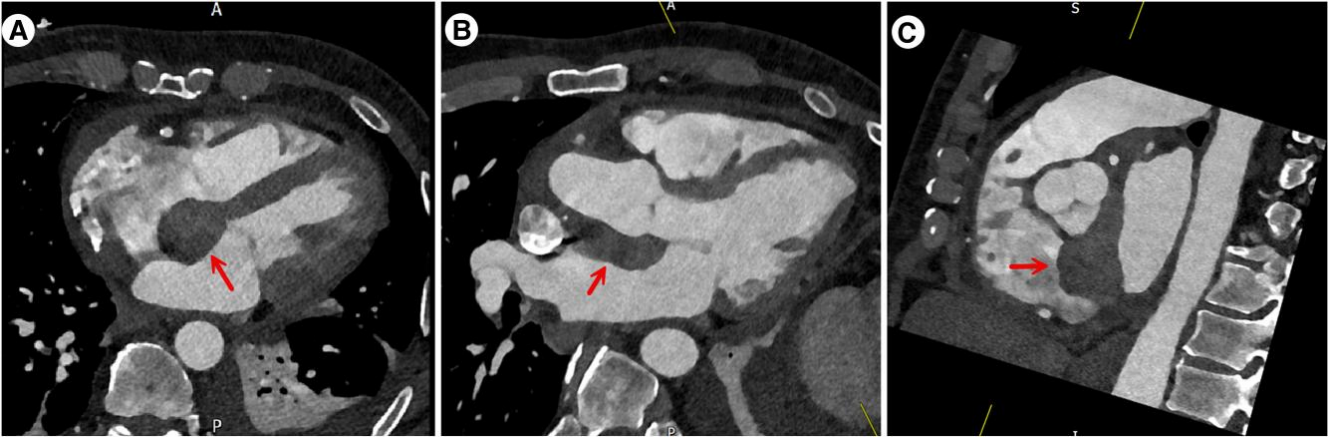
Cardiac CT scan. (*A*) Four chamber diastolic view, the red arrow highlights the mass in the atrial septum protruding into the left and right atria. (*B*) Modified axial slice showing the left ventricular outflow tract and aorta. The red arrow highlights the septal mass position in relation to the aortic root *C*. Modified short-axis view across the base of the heart shows the atrial septal mass (red arrow) association with the noncoronary and right coronary cusps.
